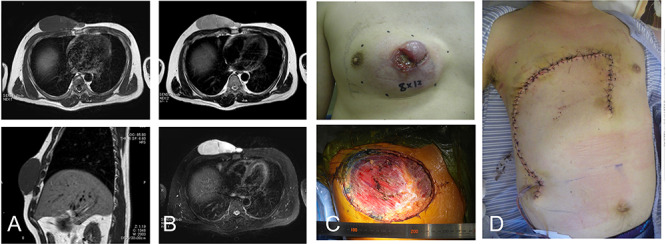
42-year-old male with dermatofibrosarcoma protuberans in the chest wall. Magnetic resonance imaging shows the subcutaneous tumor with low-signal intensity on the T1-weighted image (A) and slightly high-signal intensity on the T2-weighted image (B-upper) and high-signal intensity on a T2-weighted fat-suppressed image (B-lower); photograph of ulcerated subcutaneous lesion with subcutaneous extension; after resection of the tumor with the surrounding tissue (C), the defect was reconstructed with a thoracoabdominal flap (D).
